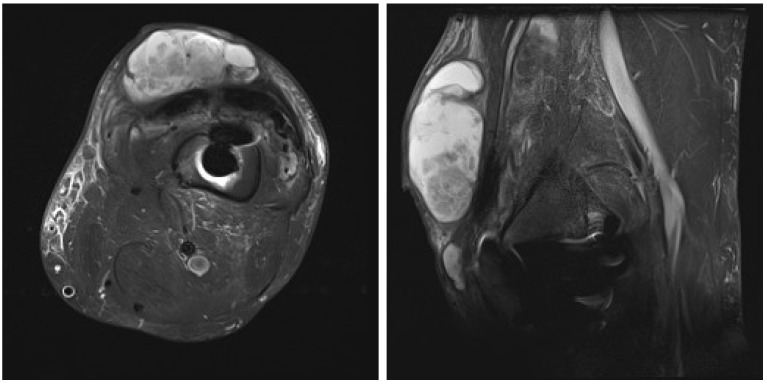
Contrast-enhanced magnetic resonance image of left knee: axial and lateral view of the left knee showing pronounced synovitis intra-articular with a small effusion, extending through the defect of the quadriceps tendon just above the patella into the subcutaneous tissue suprapatellar, forming a large septate collection, small osteolysis around the femoral part of knee endoprosthesis.
